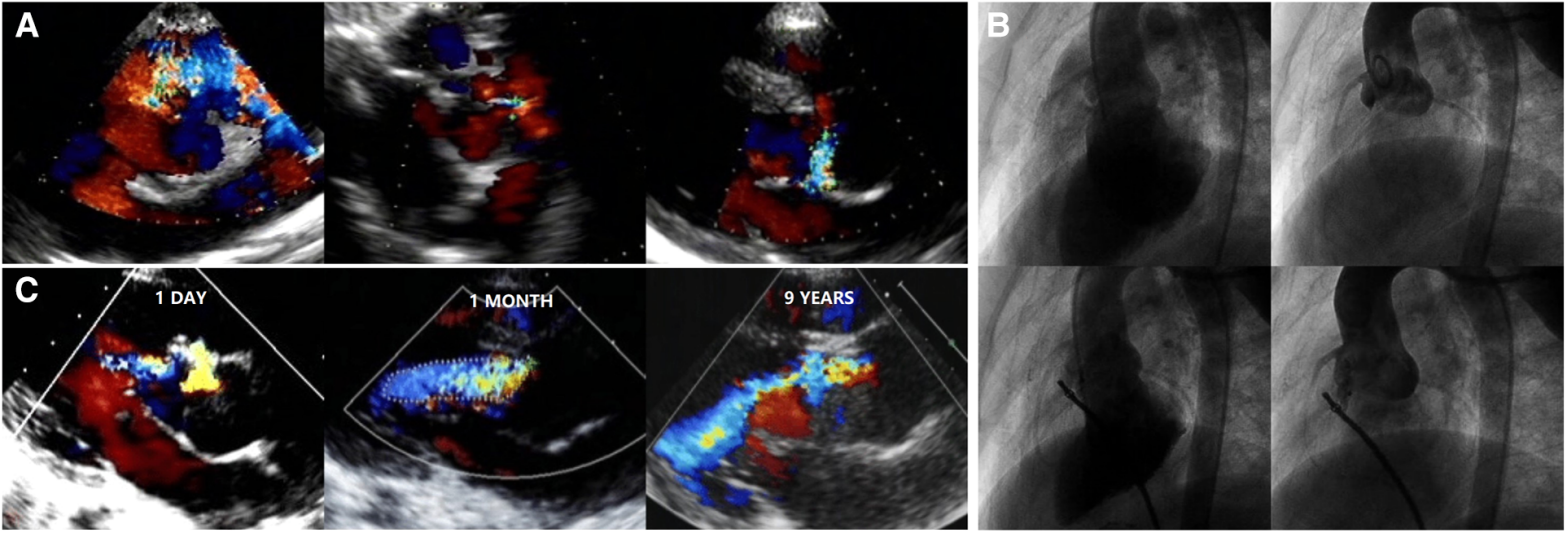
TTE and angiography in an icVSD case using a symmetrical VSD occluder: (A) features of the icVSD on TTE. (B) Features of the icVSD on angiography and a symmetric VSD occluder was used to close it with no obvious AR progression instantly. (C) AR remained mild at 1 day and progressed to moderate at 1 month and 9 years after the VSD occlusion. TTE, transthoracic echocardiography; VSD, ventricular septal defect; AR, aortic regurgitation.
